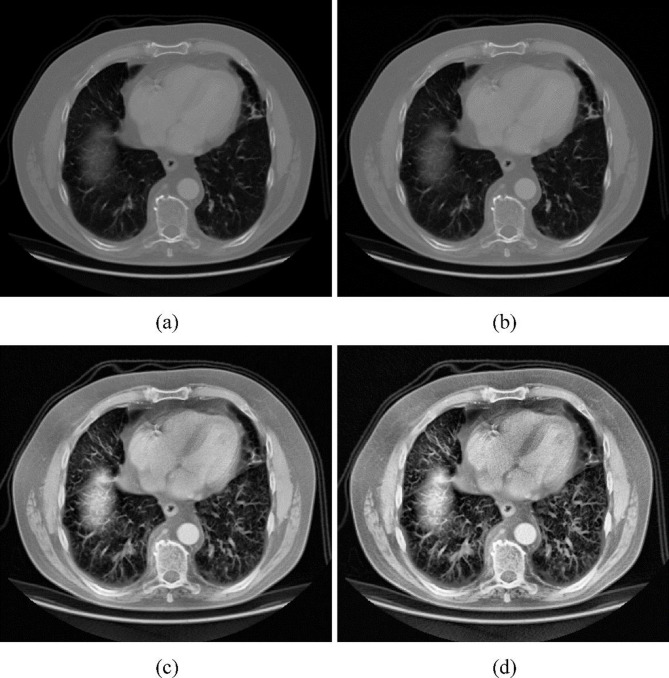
Impact of the selection of CL on the quality of an enhanced CT slice produced by the CLAHE (a) Low-contrast CT slice (b) CLAHE output (O/P) at CL = 0.0001 (c) CLAHE O/P at CL = 0.01 (d) CLAHE O/P at CL = 0.02.
